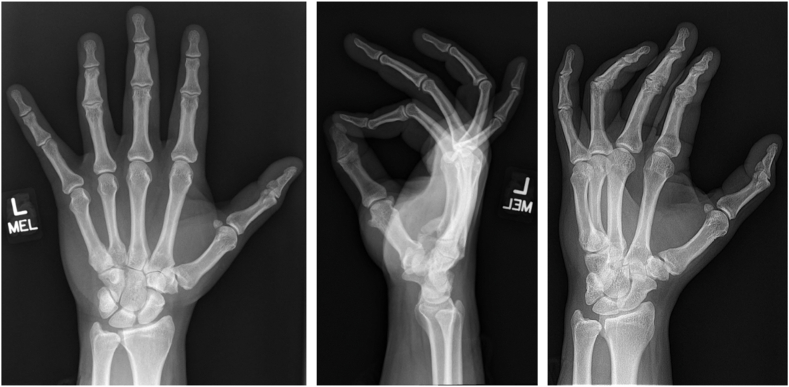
Posteroanterior, lateral, and oblique radiographs of the left hand obtained 2 months after injury demonstrating heterotopic bone arising from the volar base of the little finger metacarpal extending proximally toward the hook of hamate. There is also a small avulsion fracture at the volar base of the middle phalanx of the middle finger and evidence of a remote thumb metacarpophalangeal joint radial collateral ligament injury.
